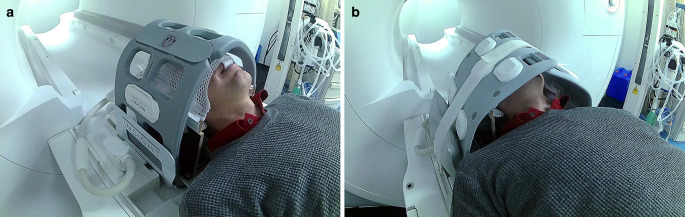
Setup for MR imaging in treatment position for brain tumor patients. a Vendor-provided setup with two four-channel Flex coils, b Our novel proposed setup with two 18-channel UltraFlex coils. In both setups, the coils are wrapped around the head of the patient, closing under the tabletop and above the nose of the patient. The most notable differences are the fixation and connection of the coils. The mask holder is a self-built wooden replica of the metallic one used during irradiation
